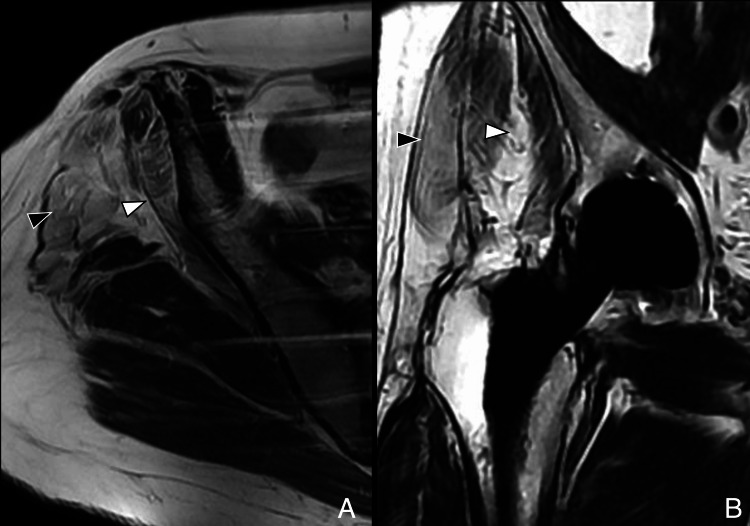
Abductor insufficiency (A) Axial T2-weighted MRI showing diffuse gluteus minimus atrophy (white arrowhead) and anterior gluteus medius atrophy (black arrowhead). (B) Coronal MAVRIC sequence in another patient shows gluteus medius (black arrowhead) and gluteus minimus (white arrowhead) atrophy following lateral approach. Image Credit: Juan Pablo Muñoz, corresponding author MAVRIC: multi-acquisition variable resonance image combination
